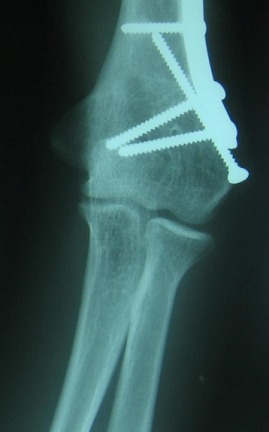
Radiographie de face montrant la correction de la fixation par vissage et plaque vissée
